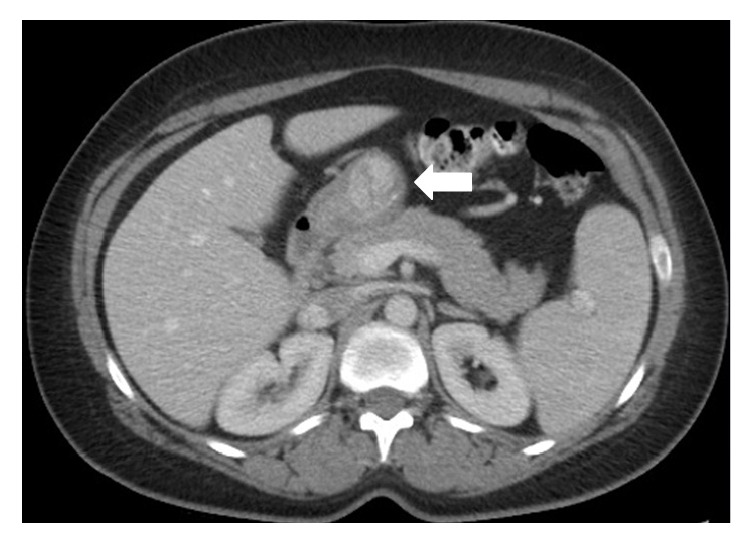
CT image of glomus tumor. The arrow is pointing to a hyperenhancing, prepyloric, submucosal mass.
